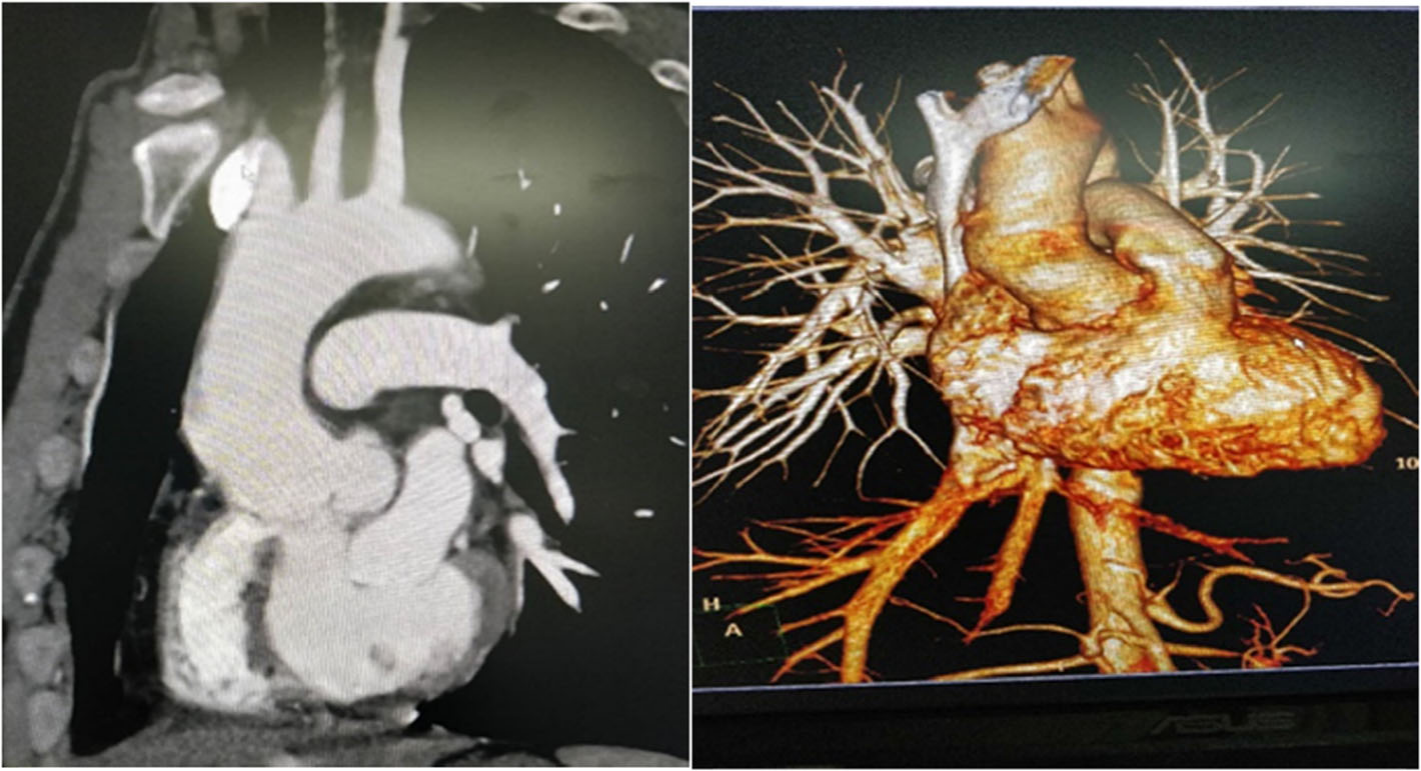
CTA scan indicated the aortic sinus is cystic outwards
